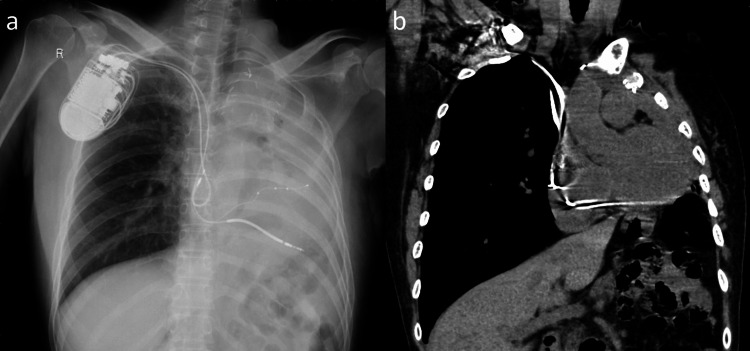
A chest radiograph (a) and a computed tomography (b) showed negligible permeability in the left lung field owing to postoperative effects, and a cardiac resynchronization therapy defibrillator was implanted in the right anterior thoracic region. There was no pleural effusion.
